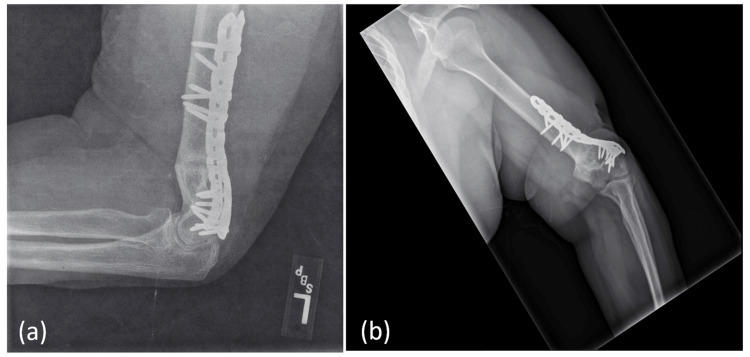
(a) X-ray image three months and (b) image 11 months post-procedure demonstrating complete fracture healing
